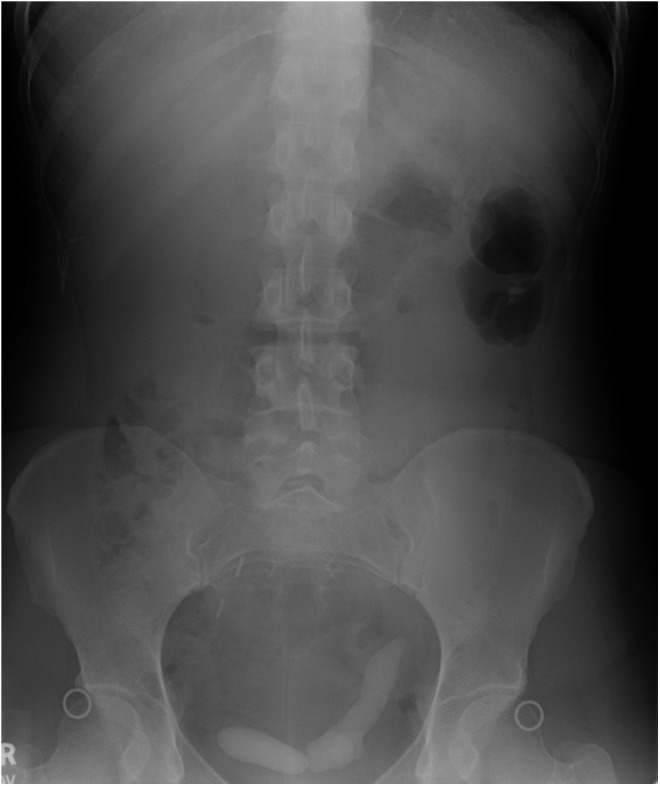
Kidney, ureter, and bladder radiograph from initial presentation.
